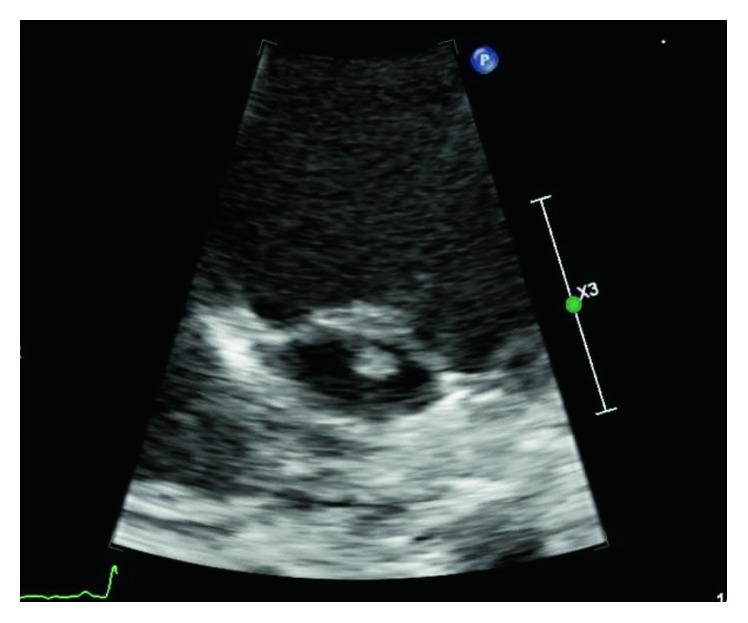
Small 3 × 5 mm vegetation on atrial aspect of the mitral valve as visualized on echocardiogram Apical four chamber view with apex up and posterior angulation.
